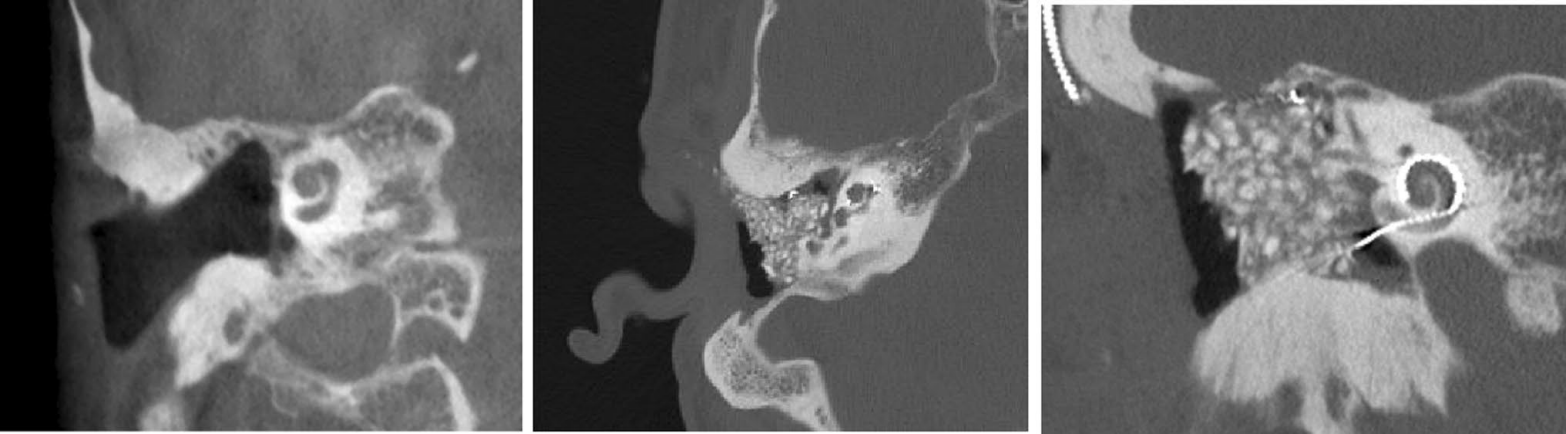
Coronal CBCT of the petrous bone of patient 4 (as an example of the surgical procedures in patients 3 and 4), a before surgery, b after surgery
